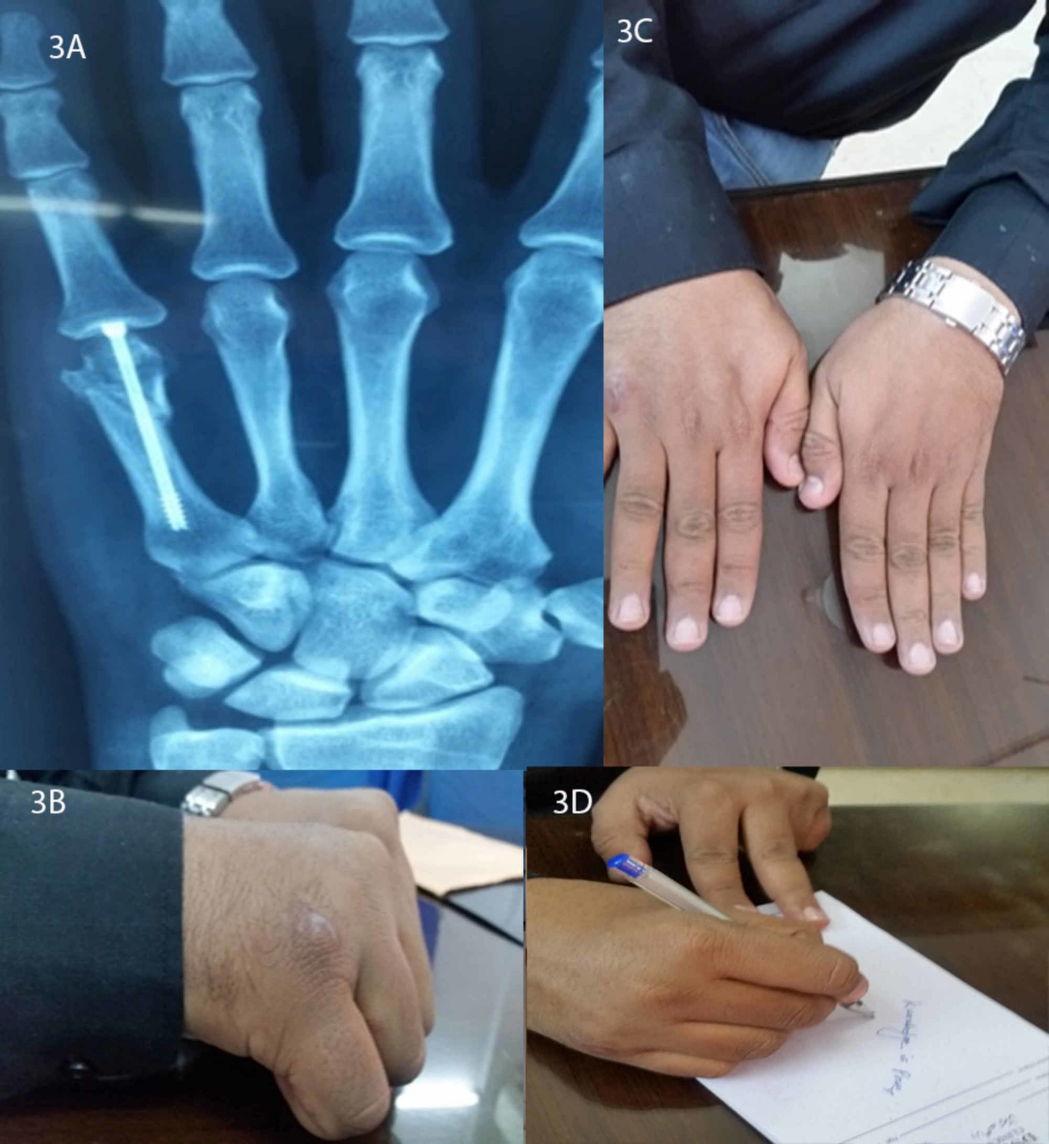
Follow-up presentation of one of the patients with a radiographic presentation (3A) and live views (3B-3D)
